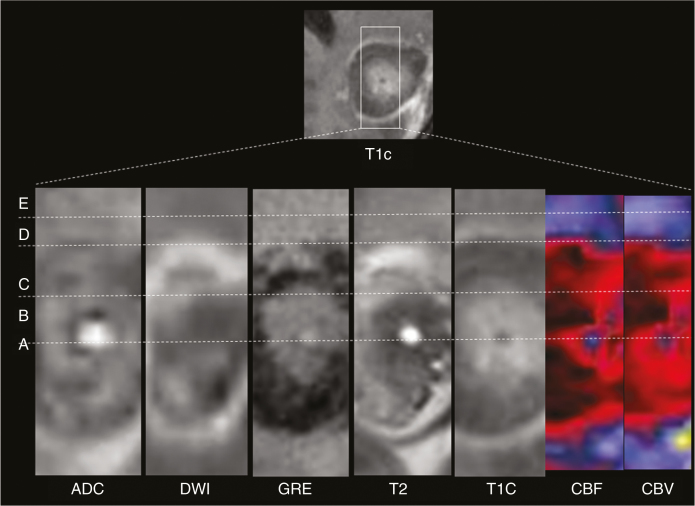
Concentric perilesional zones following LITT: ADC map, DWI, GRE, T2, T1 postcontrast (T1C), blood flow (CBF), and blood volume (CBV) images obtained 3 weeks after LITT ablation of a left parietal lesion in a 55-year-old male patient with recurrent GBM. These images demonstrate a lesion comprising five concentric zones: (A) probe track, (B) central zone, (C) peripheral zone, (D) peripherally enhancing rim, and (E) marginal zone (edema). Note that the concentric zones appear as inverse images on T1C and T2 images. Perfusion images (blood flow (CBF) and blood volume (CBV) demonstrate decreased blood volume in the marginal zone and in the peripherally enhancing rim.
